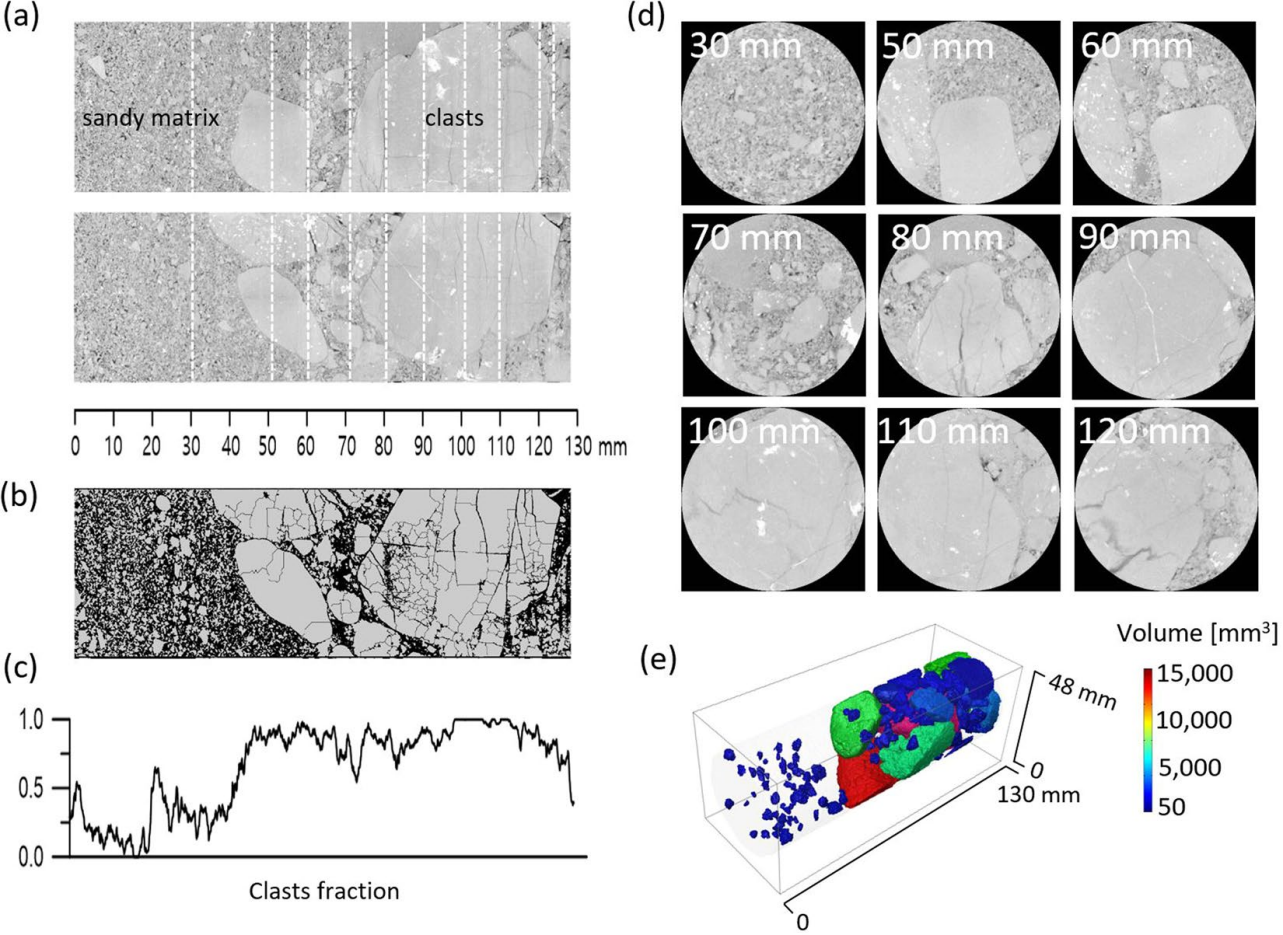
(a) Two CT cross-sectional images along the longitudinal axis taken from different angles. (b) Binary image of the core-plug for clarification of the discontinuities in the clasts. (c) The profile for clasts fraction along the longitudinal core-axis. (d) 2D cross-section images along the white-dashed lines shown in (a). (e) 3D distribution of clasts in the core-plug.
